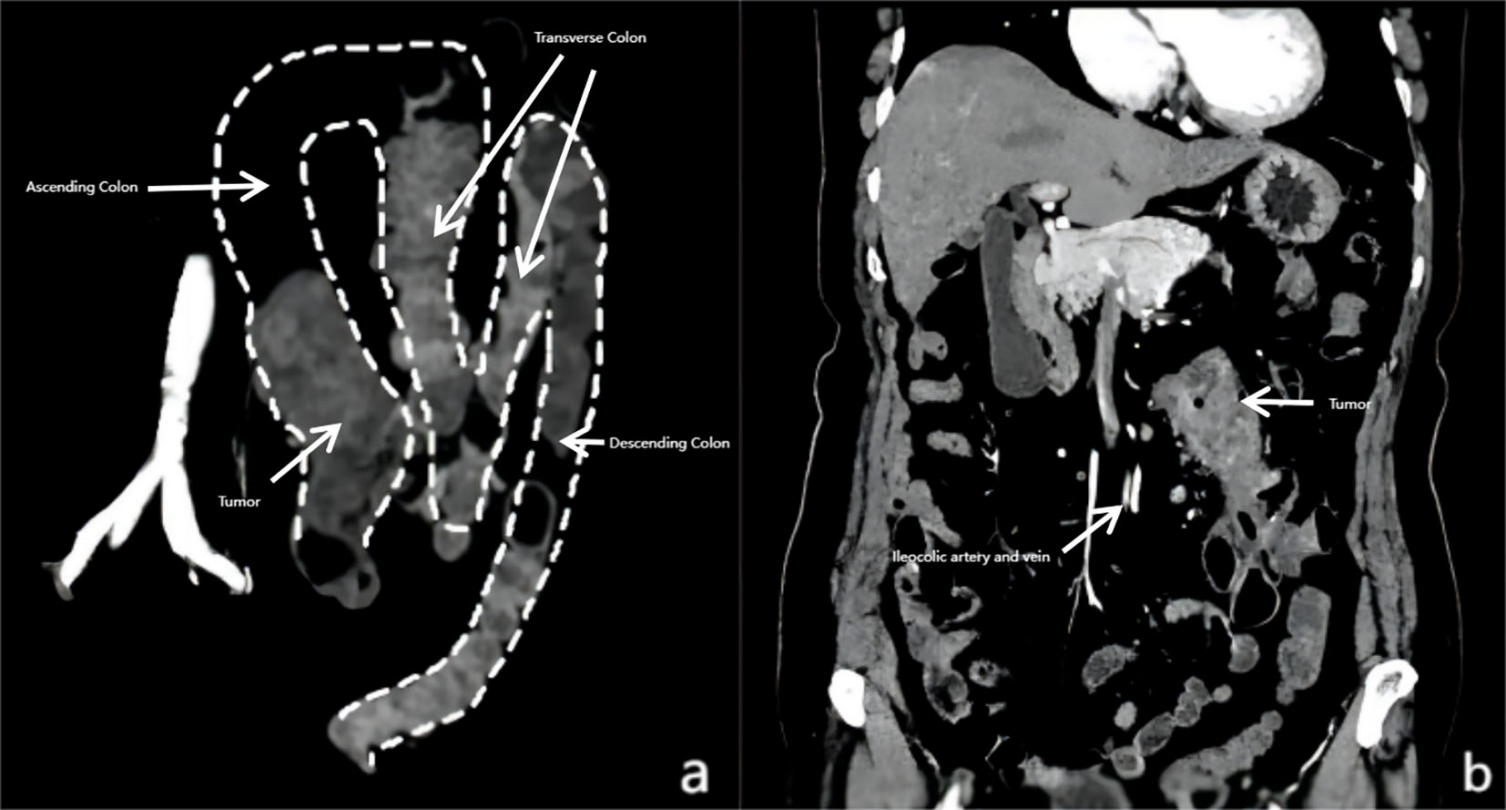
Computed tomography (CT) scan showing colorectal cancer with intestinal malrotation. (a) CT scan showing the cecum and right colon located on the left side of the aorta, forming an “M” shape due to dense adhesions among the transverse, ascending, and descending colon. (b) The ileocolic artery and vein originating from the left side of the superior mesenteric artery (SMA), which were positioned to the left of the abdominal aorta.
